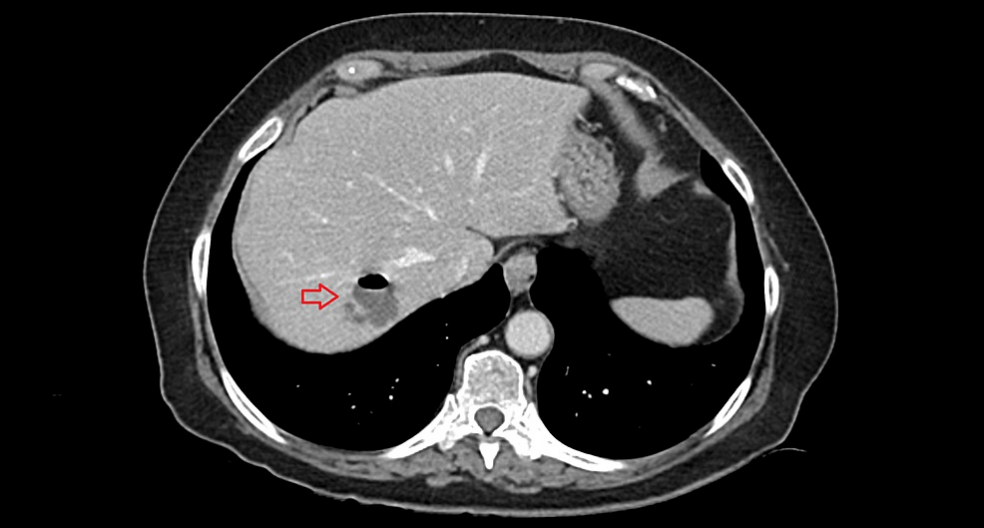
Abdominal CT showing a liver abscess (red arrow) CT: computed tomography
